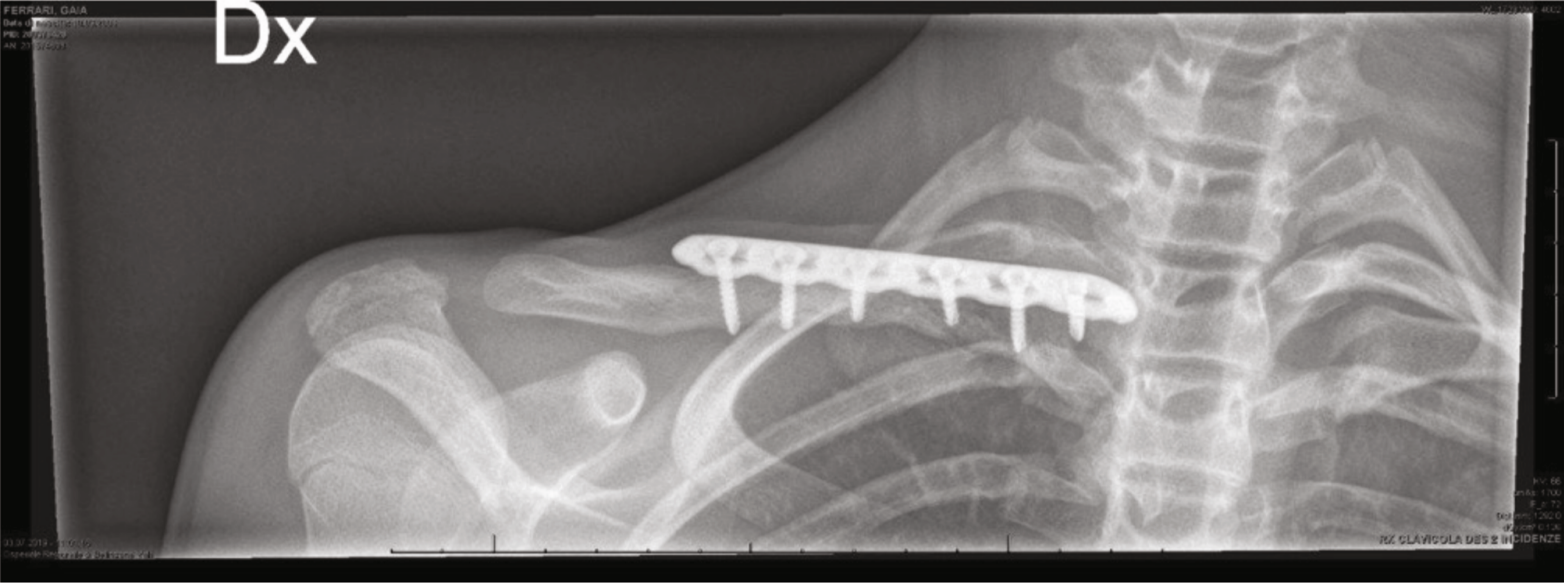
X-ray imaging at 1 year.
